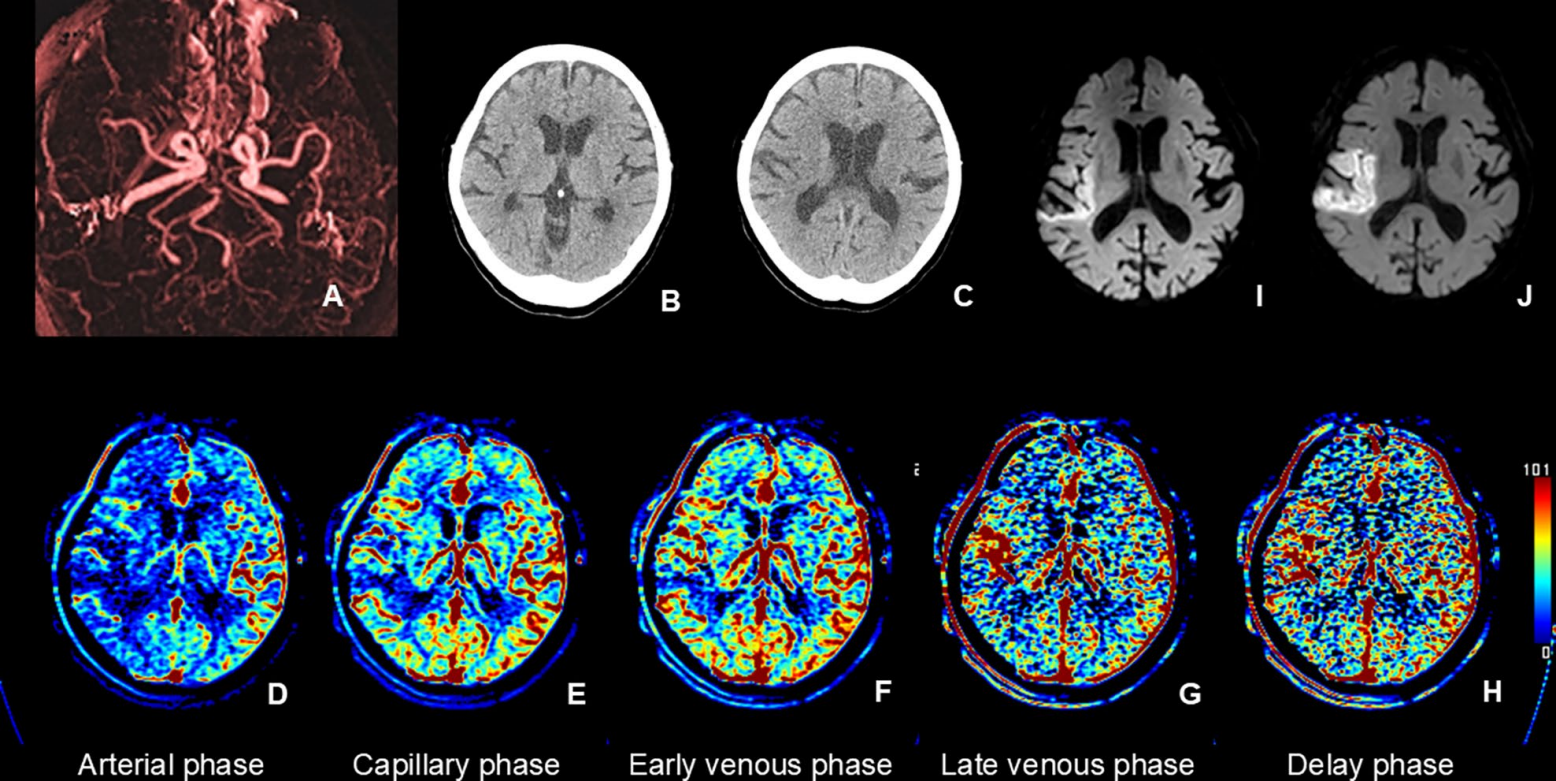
Images of an 87-year-old woman with occlusion of the right middle cerebral artery (MCA) demonstrated on CT angiography (A) derived from CT perfusion. The patient had a premorbid modified Rankin scale score of 0 and presented with the National Institutes of Health Stroke Scale score of 9 upon admission. Conservative treatment was administered. At 90 days, the patient's mRS score was 3. Initial brain CT images (B and C) showed ambiguous differentiation between the cortical gray matter and white matter in the right insula, making it difficult to accurately determine the extent of the baseline infarct. The CT collateral map (D–H), generated from the admission CT perfusion, indicated a moderate collateral perfusion status (collateral perfusion score of 3: collateral perfusion delay affecting less than half of the middle cerebral artery territory during the capillary and early venous phases), suggesting potential infarct growth. Diffusion-weighted imaging (DWI) (I), performed immediately after the CT scan, showed acute infarct signals within the right MCA territory. The extent of the DWI lesion at admission (I) corresponded closely with the hypoperfused area observed in the early venous phase (F) of the CT collateral map. On Day 1, follow-up DWI (J) revealed that the baseline lesion had expanded to a size similar to the hypoperfused area seen in the capillary phase (E) of the CT collateral map at admission. These findings suggest that even in the absence of DWI, the CT collateral map could potentially be used to identify the baseline lesion and predict infarct growth, as well as estimate the potential expansion of the infarcted area
